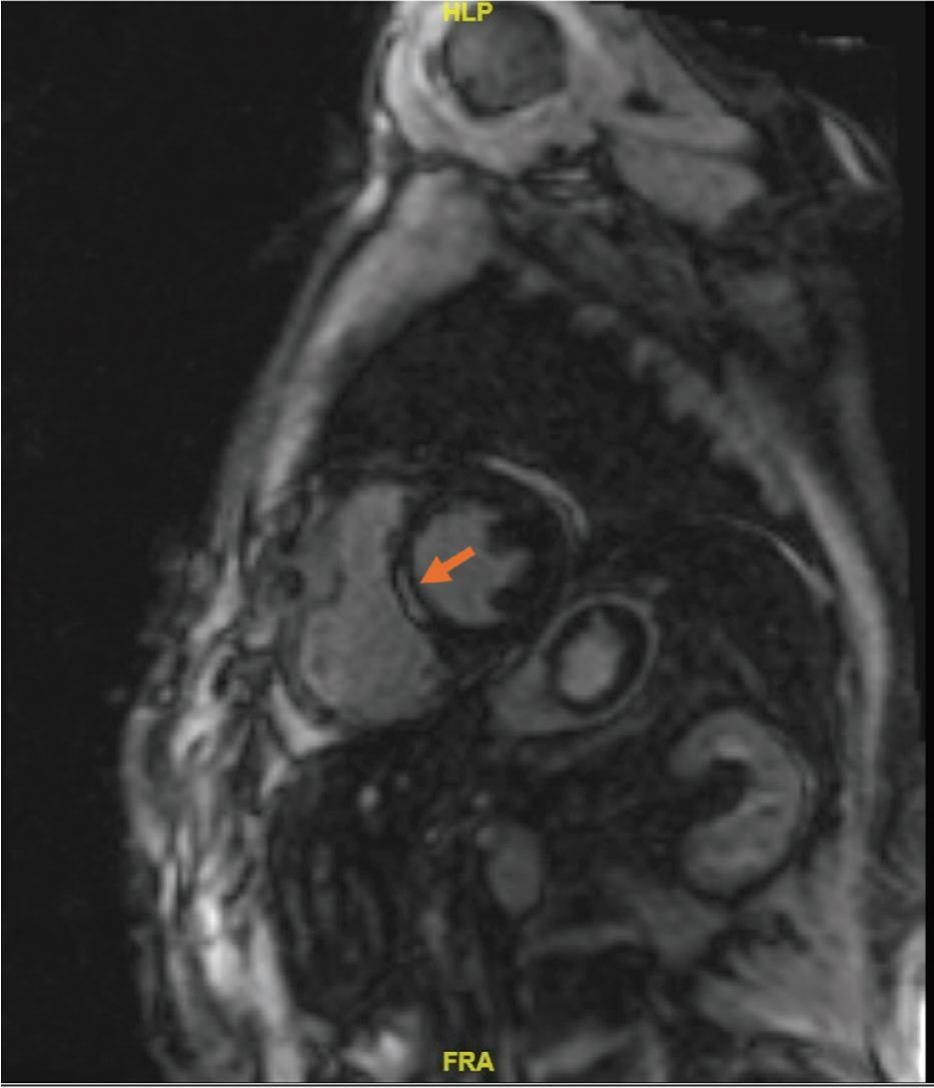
Cardiac magnetic resonance imaging MRI shows isolated late enhancement in the area of the interventricular septum, typically observed on T1‐weighted images.
